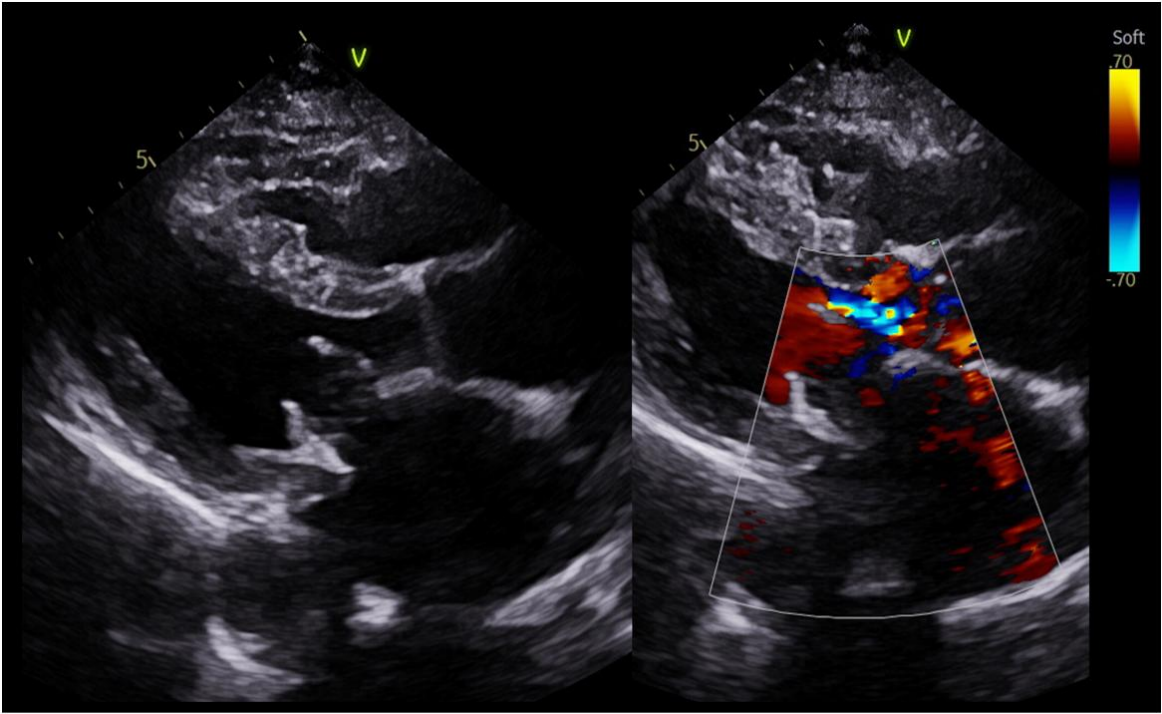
Echocardiography. Parasternal long axis demonstrating crowding of the LVOT with chordal SAM secondary to native anterior mitral valve leaflet. Flow acceleration within the LVOT secondary to Edwards S3 Ultra within 30 mm Physio ring (26 mm).
